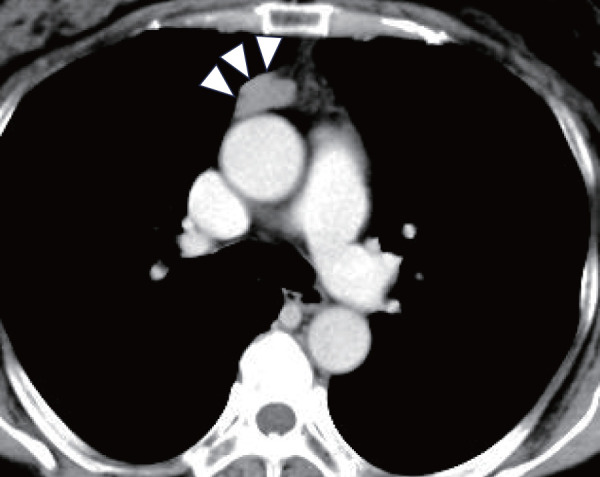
Contrast-enhanced chest CT demonstrating a lobulated cystic mass (23 × 14 × 33 mm) in the anterior mediastinum, broadly contacting the pericardium. The lesion exhibits homogeneous internal density with minimal contrast enhancement, raising suspicion for a thymic or pericardial cyst (arrows). CT: computed tomography
